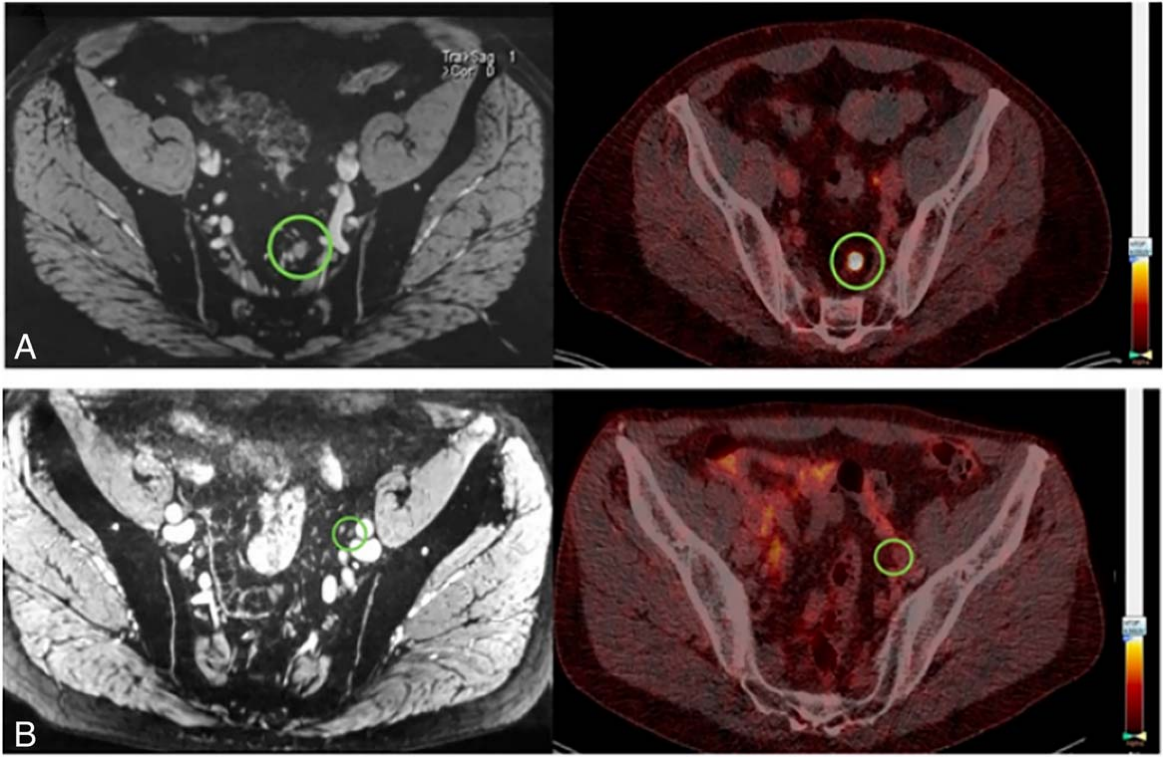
MRI and PSMA-PET/CT images from a patient with metastatic PCa. Iron-sensitive T2*w fat-saturated USPIO-enhanced MRI (left) and the corresponding fused PSMA-PET/CT scans both identify a 7-mm lymph node in the left pararectal region (green circle in A). A 4-mm lymph node adjacent to the left external iliac artery is positive on MRI only, but remains undetected on PSMA-PET/CT (green circle in B). Partially reproduced with permission from Schilham et al.^68^
